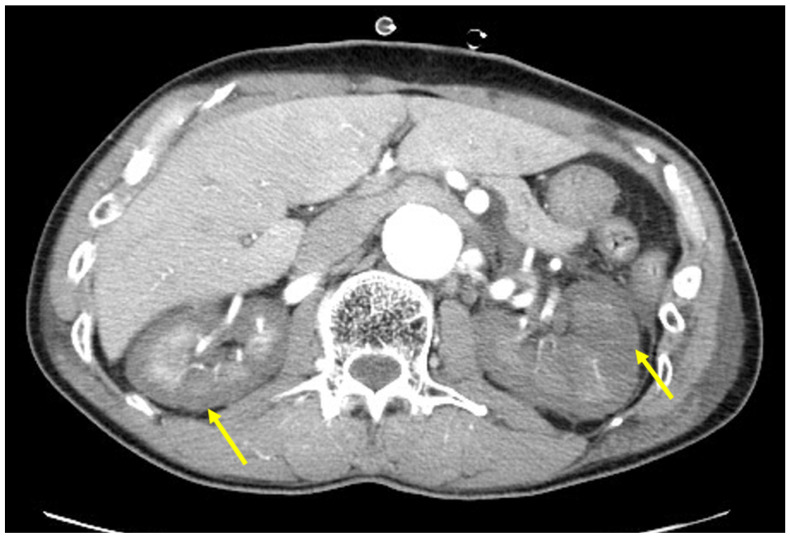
Contrast-enhanced abdominal CT of a t-TMA patient showing severely reduced renal perfusion (yellow arrow).
